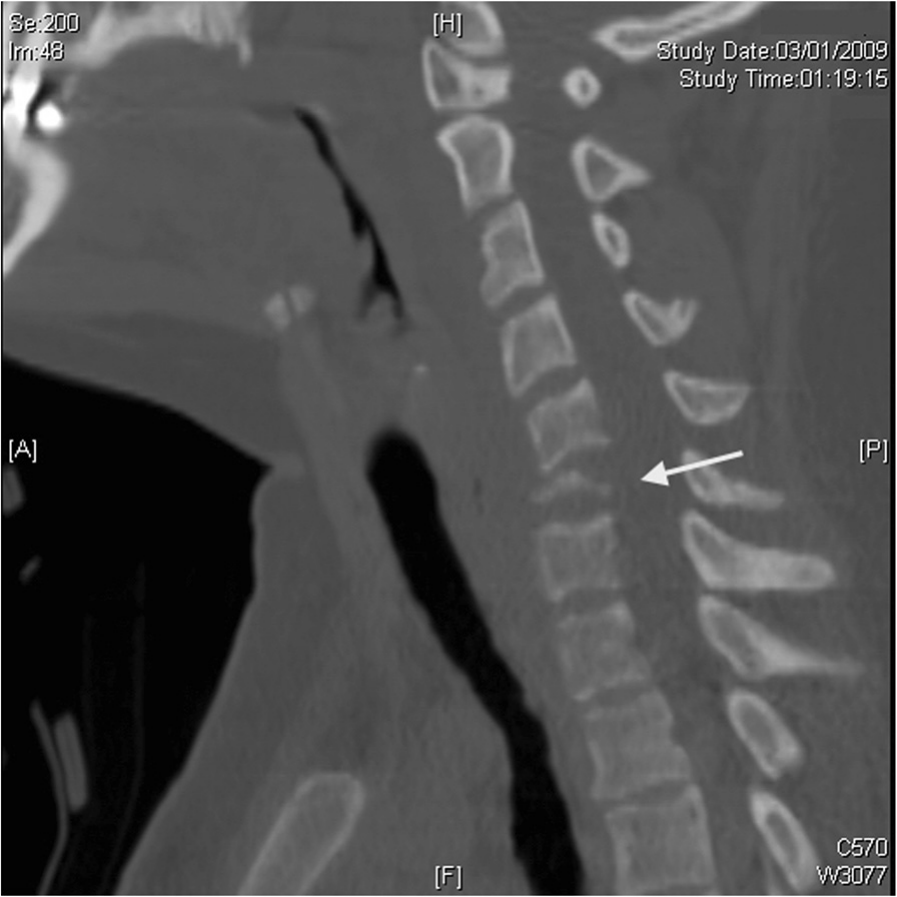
Preoperative sagittal computed tomography showing near complete destruction of C6 vertebral body (arrow)
